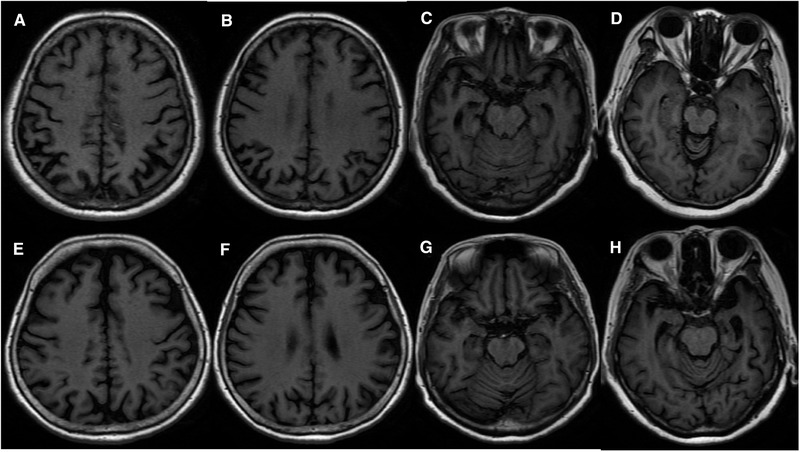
Brain MRI of 4 cases. A‐C, E‐G are from two female EOAD cases with a disease course of three years. The case with A‐C is 62 years old with ApoE genotype of ε3ε3, and the case with E‐G is 63 years old with ε4ε4. The ε3ε3 carrier shows greater cortical atrophy than the ε4ε4 carrier in parietal, occipital and temporal regions. D and H are from two female LOAD cases with a disease course of two years. They are 76 and 75 years old, respectively. ApoE genotype are ε2ε3 and ε3ε4, respectively. The ε3ε4 carrier shows greater cortical atrophy than the ε2ε3 carrier, mainly in medial temporal lobes
